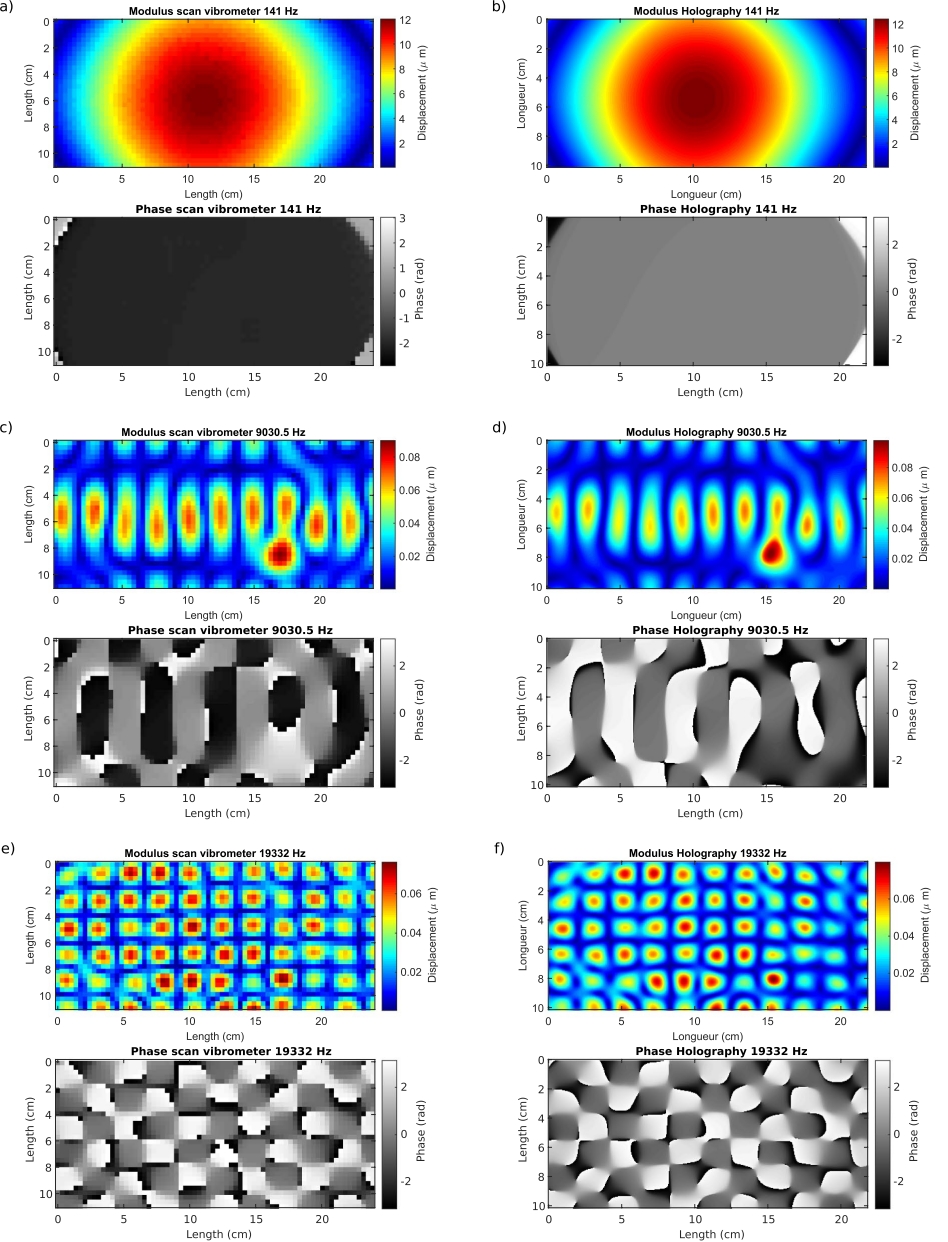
Amplitude and phase of the operational displacement fields of the structure measured with scanning point-wise laser Doppler vibrometer at (a) 141 Hz, (c) 9030.5 Hz, (e) 19332 Hz, and coherent imaging at (b) 141Hz, (d), 9030.5Hz, (f) 19332Hz.
